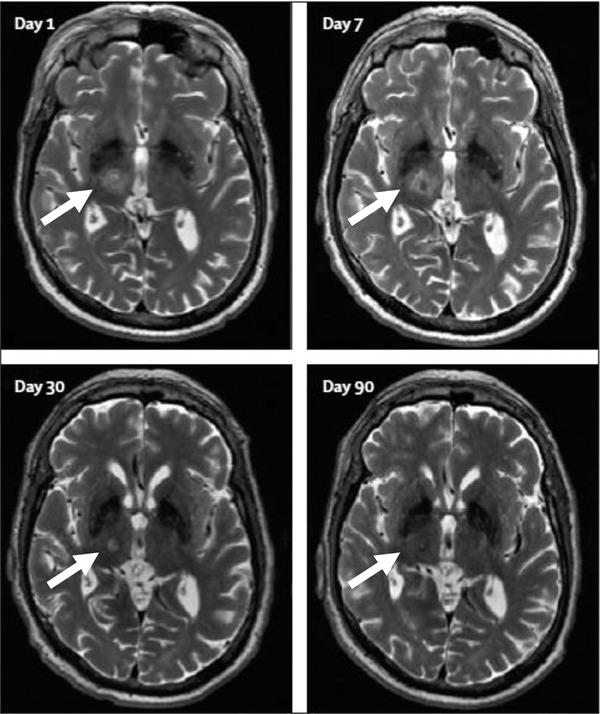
Ultrasound surgery of the right unilateral thalamus (white arrow) of a patient with essential tremor performed with a focused ultrasound helmet system (compare Figure 1). Lesion generation (day 1) and subsequent healing are shown over a period of 90 days. Adapted with permission.^[^
